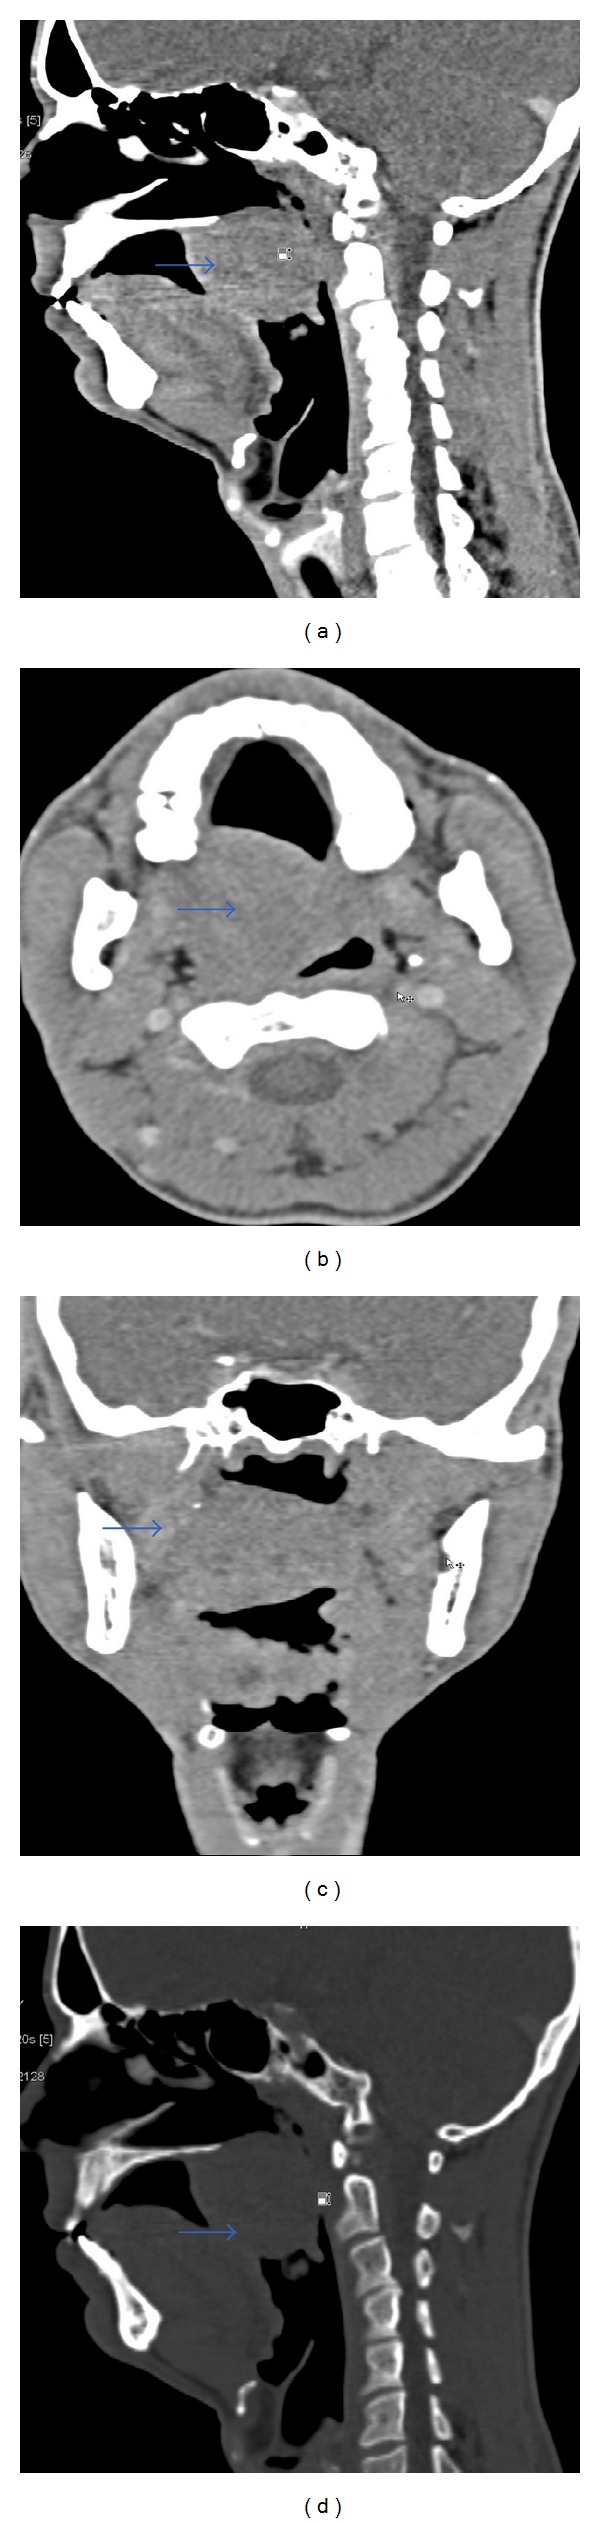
Contrast enhanced CT soft tissue window (a) sagittal section, (b) axial section, (c) coronal section, and (d) bone window showing well defined mildly enhancing heterogeneous soft tissue mass (marked by arrow) arising from soft palate reaching up to the posterior pharyngeal wall causing significant narrowing of nasopharyngeal and oropharyngeal airway. No cystic area or fat or calcification within the mass. The mass is abutting the hard plate with no erosion or destruction.
